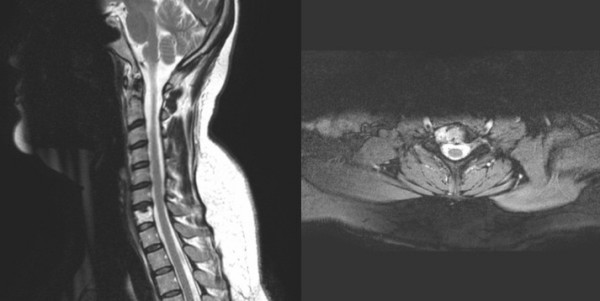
Axial and sagittal T2-weighted MRI images of cervical spine. Osteolysis of C7, compromising the stability of the vertebra.
